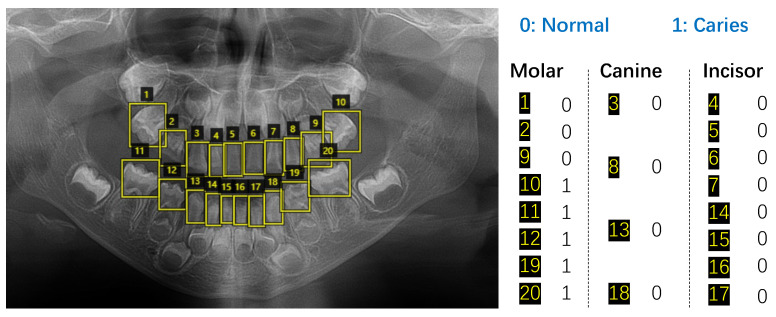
Extracting and labeling each tooth on a panoramic radiograph.
